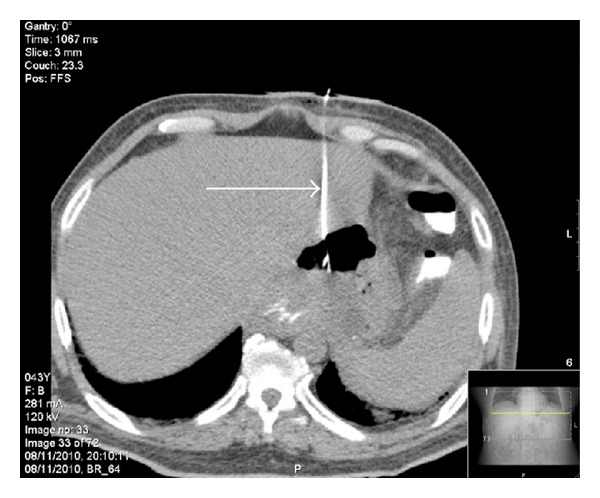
CT scan detected an abscess with dimensions of 4.9 × 9.0 cm located along the subdiaphragmatic border near the gastroesophageal junction. CT identified no gastric leak. The abscess was drained with a 7-French drainage tube (arrow).
